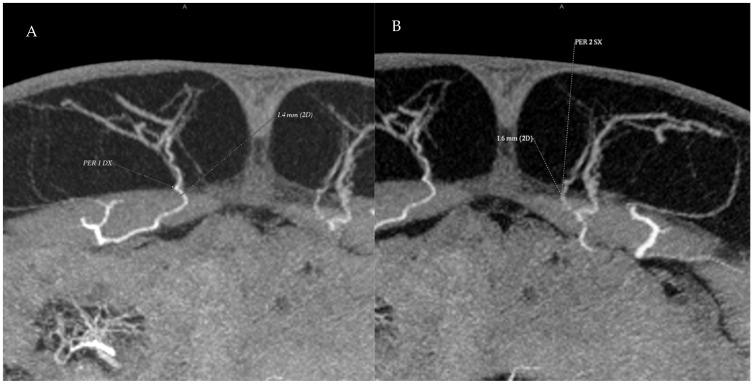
Preoperative CTA images showing the perforating vessels of the deep inferior epigastric artery in the periumbilical region. (A) A single right-side perforator with a diameter of 1.4 mm is identified. (B) Two adjacent left-side perforators are visible; the more medial perforator measures 1.8 mm in diameter.
